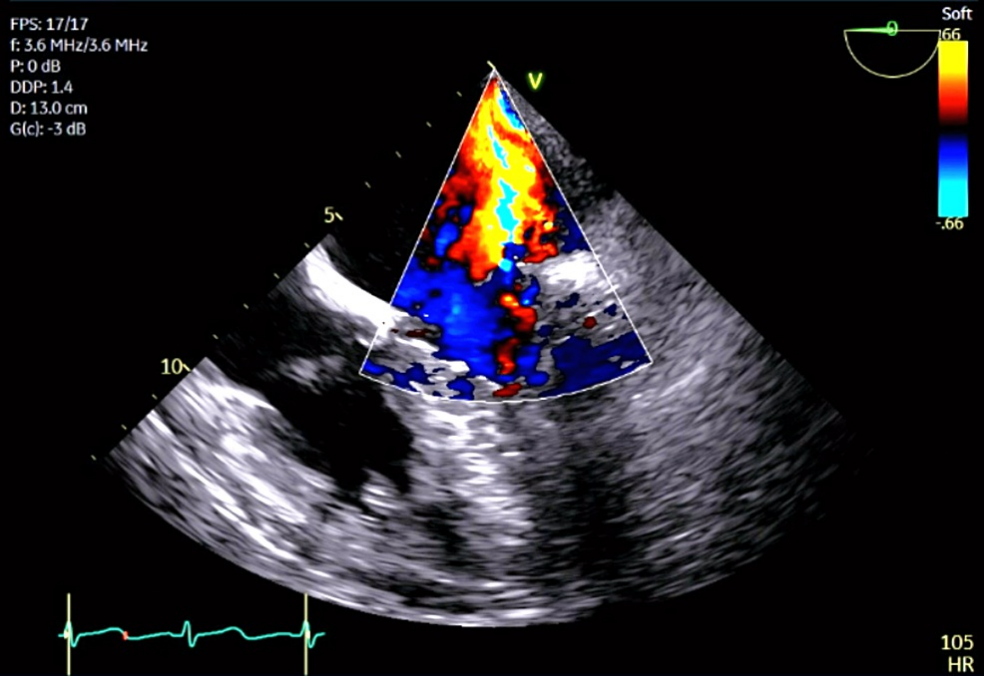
Transesophageal echocardiogram in color Doppler mode demonstrating mitral valve regurgitation.
